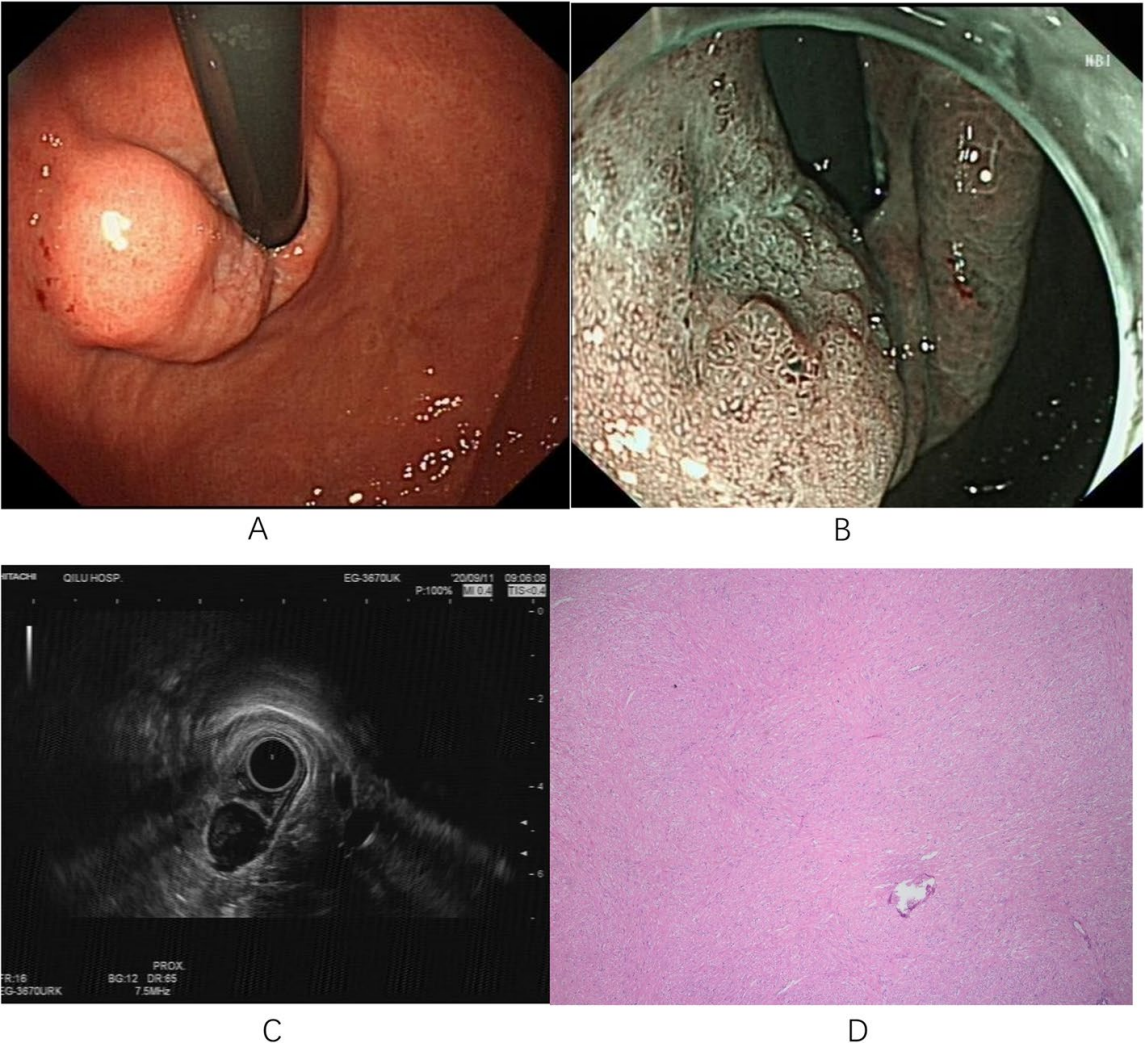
Case 4: A A 1.0x2.0cm elevated lesion on the posterior wall of the cardia with a rough mucosal surface and a slight central depression. B ME-NBI shows an increase in the microvascular diameter and irregular microglandular pattern. C Endoscopic ultrasonography scan showing a 2.0x1.6cm hypoechoic cluster with homogeneous internal echogenicity. D A leiomyoma in the submucosa (magnification 40x). ME-NBI, magnifying endoscopy with narrow-band imaging
